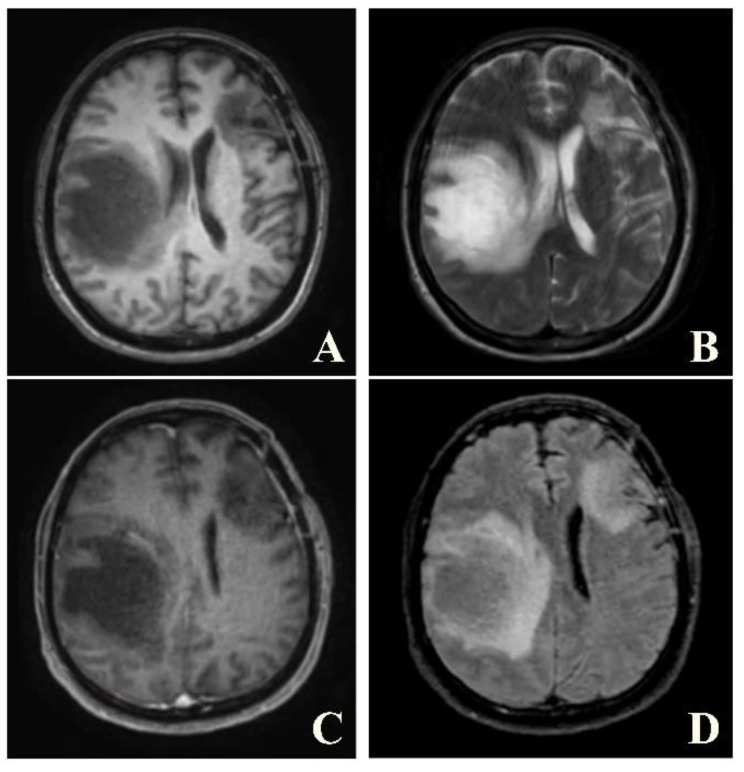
Axial T1W (A), T2W (B), post-contrast T1W (C) and post-contrast FLAIR (D) images of the same patient reveal tumor-like lesion with surrounding vasogenic edema at left frontal and right parietal lobes. The patient was suspected to have high-grade glioma and underwent biopsy. Pathological result was consisted with demyelination, yielding a final diagnosis of tumefactive MS. The patient was given treatment with high-dose steroids.
